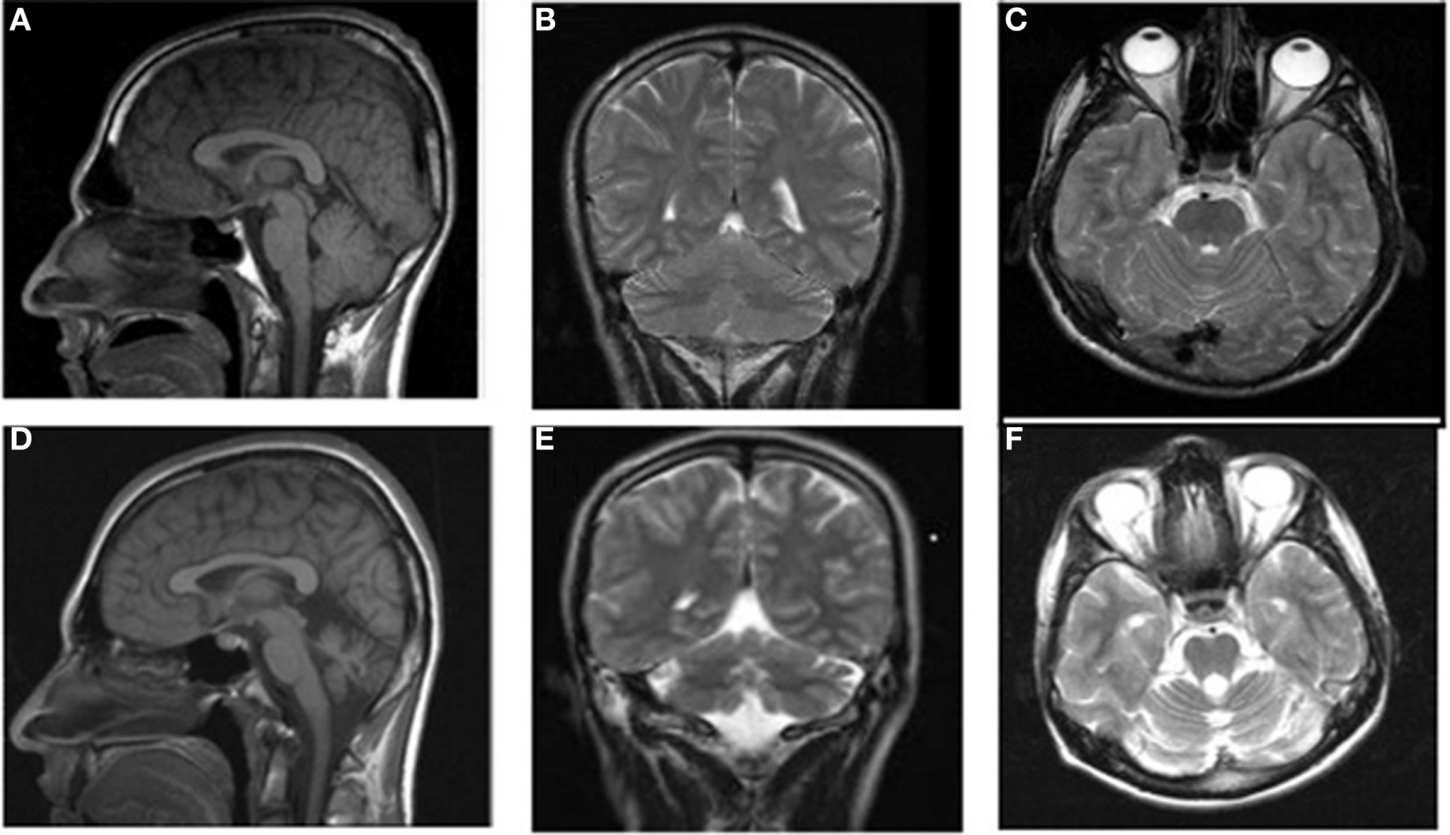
MRI scans from a healthy subject: sagittal (A), coronal (B), and axial (C) and the patient IV-24: sagittal (D), coronal (E), and axial (F).
